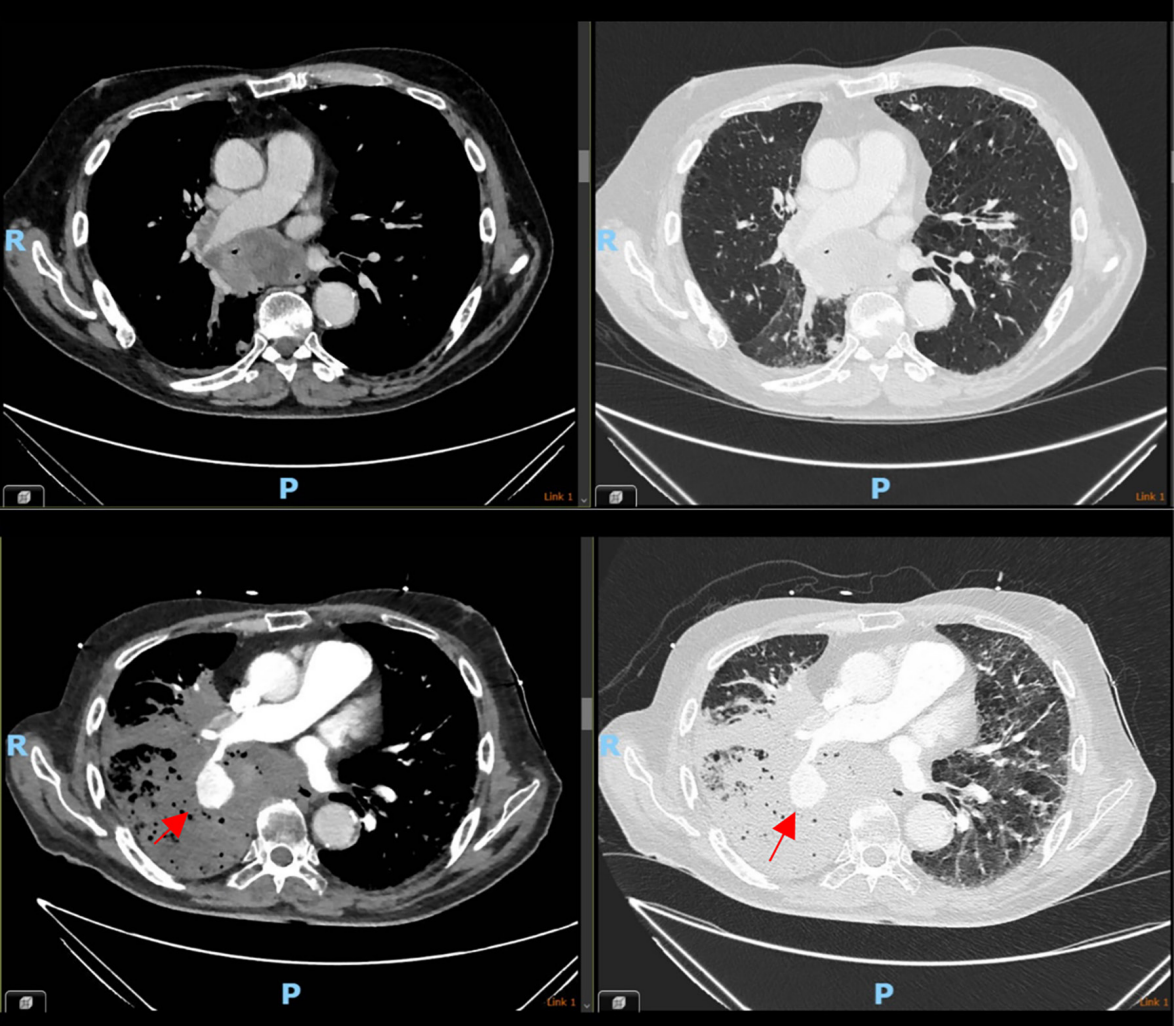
Comparison between the CT chest of the patient in February vs. April, demonstrating a new large pulmonary artery pseudoaneurysm.
